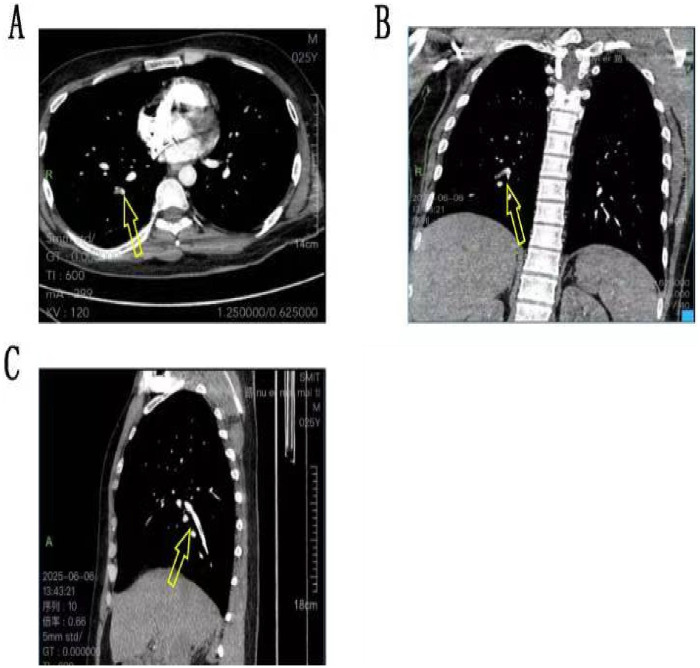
Combined pulmonary artery CTA. (A–C) Contrast agent filling in the pulmonary artery lumen is not uniform (indicated by arrows) that suggesting a possible localized pulmonary embolism.
